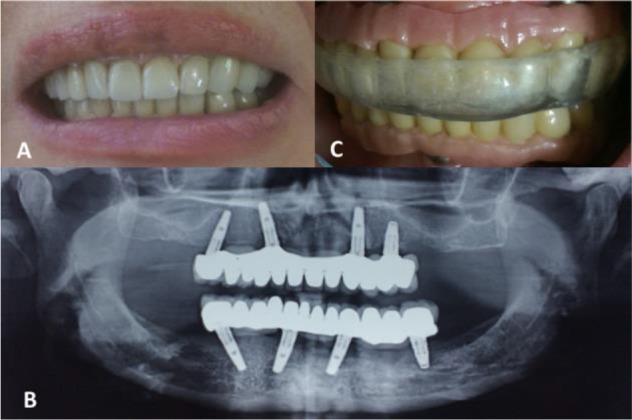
(A) Clinical and (B) radiographic views of metal-ceramic crowns cemented on metal mesostructures. (C) A maxillary night guard for lowering the risk of porcelain fracture
